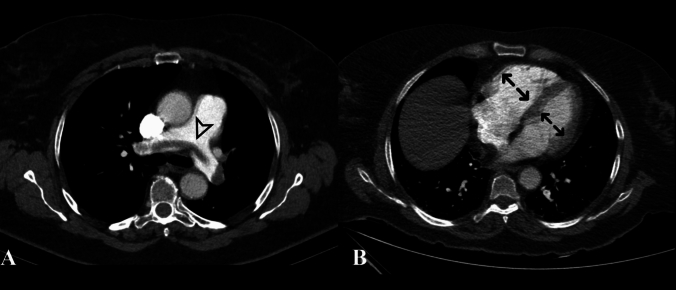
Axial CT pulmonary angiography (CTPA) demonstrating a saddle thrombus straddling the main pulmonary artery (white arrow, panel A). Panel B shows measurement of the right ventricle-to-left ventricle (RV/LV) diameter ratio (black arrows), an indicator of right ventricular strain if > 0.9
